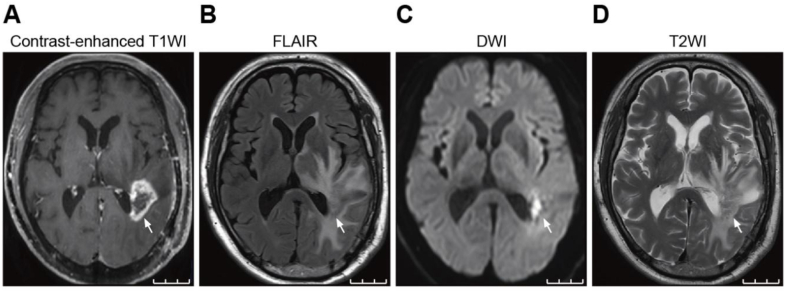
MRI of the brain lesions before treatment with bevacizumab. Contrast enhanced T1-weighted image (T1WI) (A), T2-weighted fluid-attenuated inversion recovery (FLAIR) image (B), and diffusion-weighted image (DWI) (C) acquired prior to bevacizumab treatment are shown. Contrast-enhanced T1WI shows an irregular enhancing lesion protruding into the excisional cavity in the left temporal lobe (arrows). The lesion exhibits high signal on DWI, low signal on the ADC map, and surrounding FLAIR hyperintensity. Scale bars: 30 mm.
